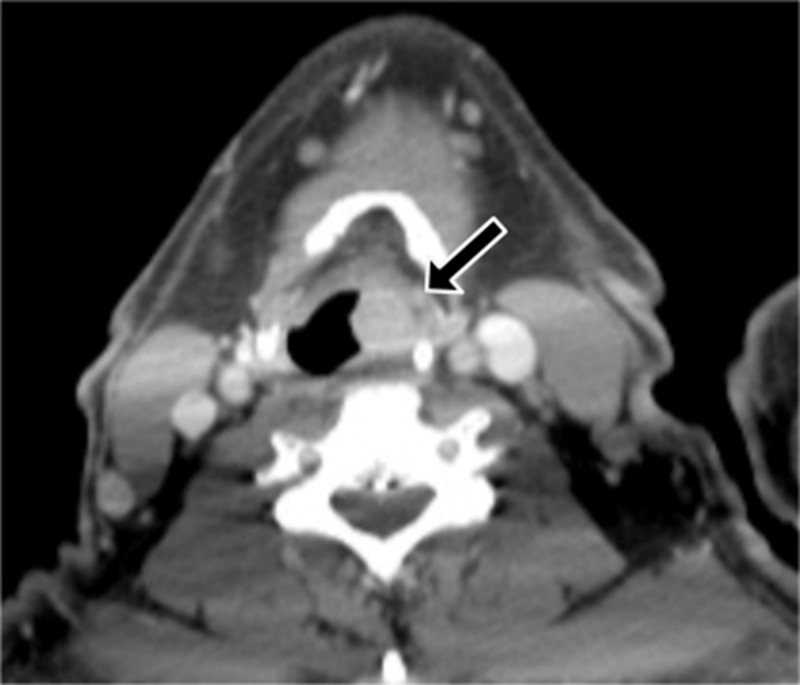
Axial CT image demonstrating a well-defined enhanced nodule (black arrow) in the left pyriform sinus.
